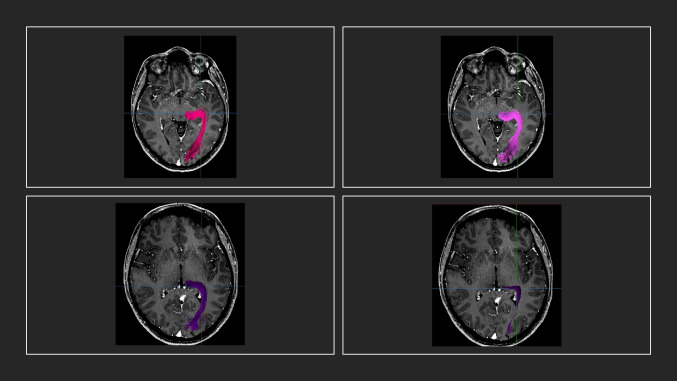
MRI T1-weighted + gadolinium in axial view displaying a temporopolar tumor in the proximity of the OR. Upper line/rater 1: left: OR based on DTI-FT. Right: OR based on QBI-FT. Lower line/rater 2: left: OR based on QBI-FT. Right: OR based on DTI-FT. (MRI, magnetic resonance imaging; OR, optic radiation; QBI, Q-ball Imaging; DTI, Diffusion Tensor Imaging)
